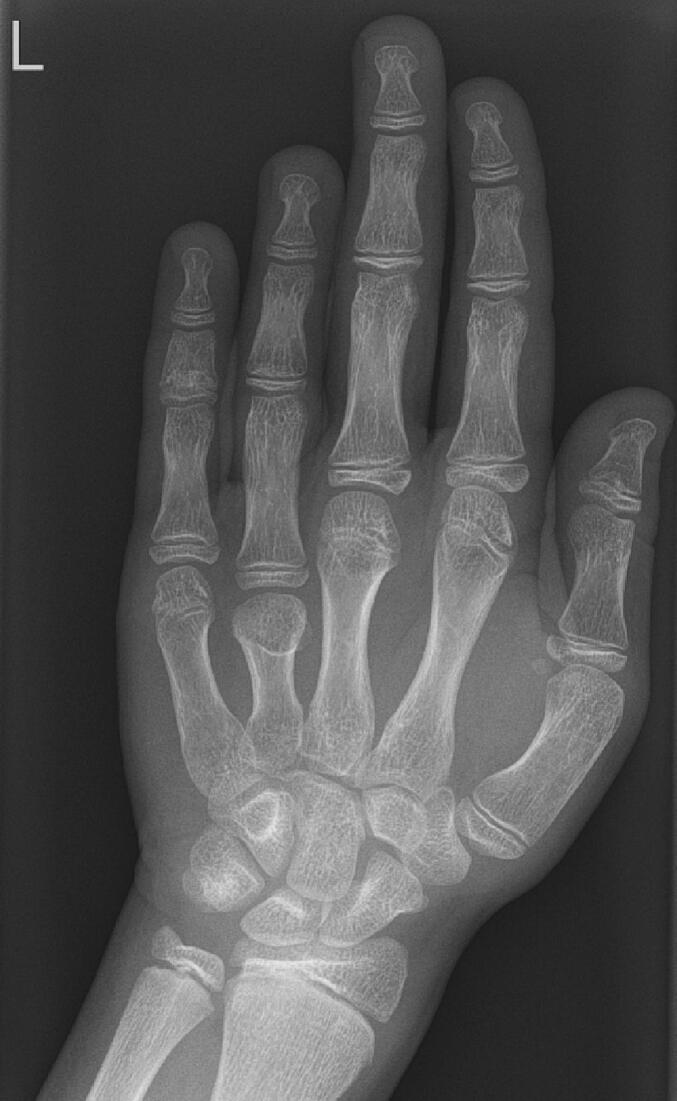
Hand X-ray of patient 1 at the age 12 years.
